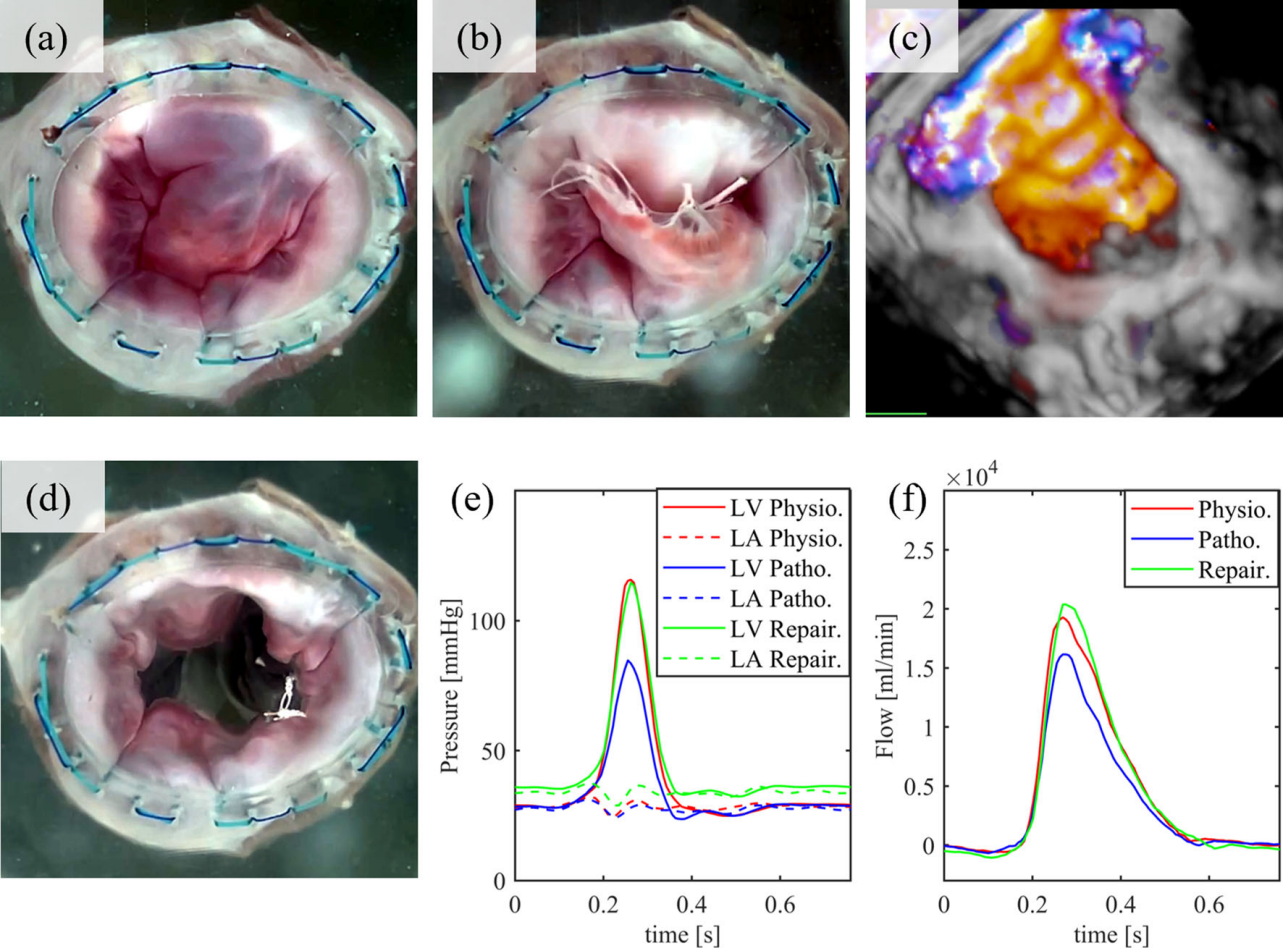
a–d Ex-vivo valve A in physiological condition (a), after chordae-rupture in pathological condition (b), 3D-color-doppler in pathological condition showing a big regurgitation jet (c), and after performing MIMVS in repaired condition (d). e, f Pressure (e) and Flow (f) graphs. LV, left ventricle; Physio., physiological condition; LA, left atrium; Patho., pathological condition; Repair., repaired condition
